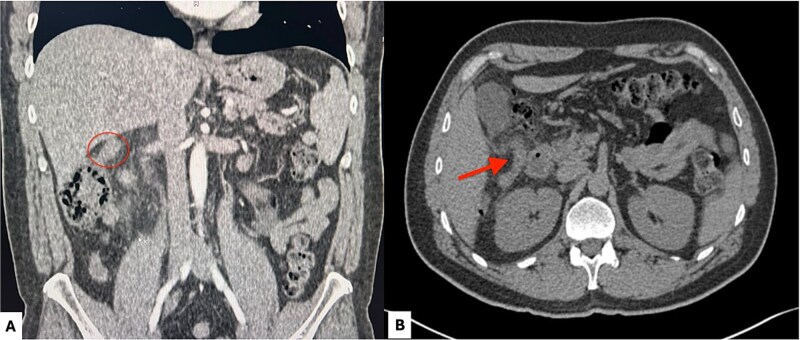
Contrast-enhanced abdominal computed tomography. (A) Coronal view showing a subhepatic appendix (red circle) measuring 12 mm in diameter. (B) Axial view demonstrating a subhepatic appendix (red arrow) with mural thickening, increased enhancement, and surrounding periappendiceal fat stranding.
